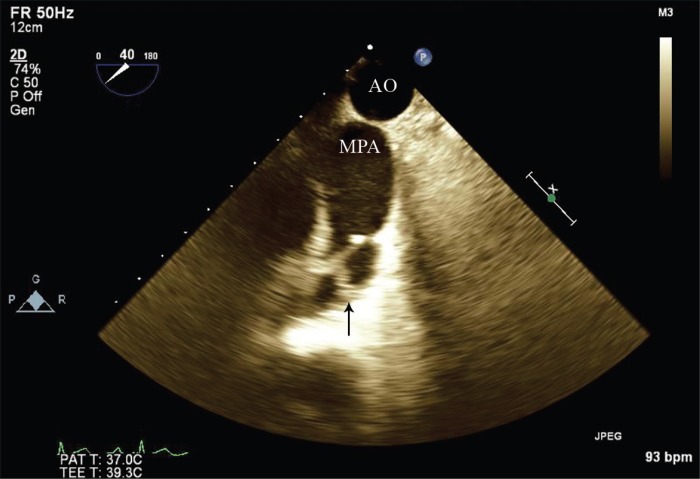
Two-dimensional transesophageal echocardiography at 40° upper esophageal view, showing that the tip of 1 pulmonary cusp is attached to the sinotubular junction (Arrow).
